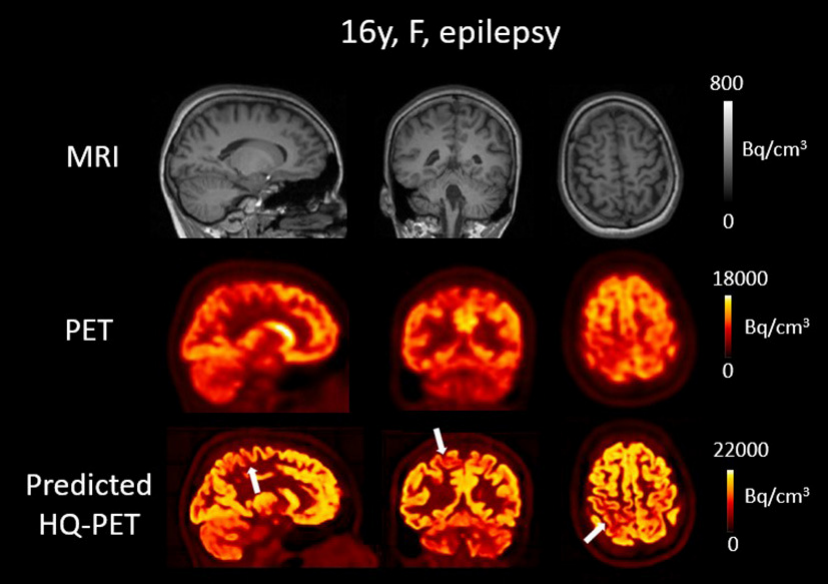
Brain T1w MRI and clinical [^18^F]FDG PET as well as predicted high-quality (HQ) PET (predicted by the network developed in this work) from one patient with drug-resistant epilepsy. Images are displayed using radiological conventions (subject's left on the right) and white arrows are used to highlight areas of hypometabolism. The first two rows show images from the scanner and the third row shows the AI-enhanced high-quality PET. MRI depicted a blurred white matter gray matter border in the right postcentral gyrus. The PET showed a correlated blurred and mild hypometabolism extending toward the precuneus. The predicted HQ PET showed a clearer hypometabolism very well correlated with the lesion that also extended to the precuneus. Bq, Becquerel *cm*^3^*: centimetres cubed*.
